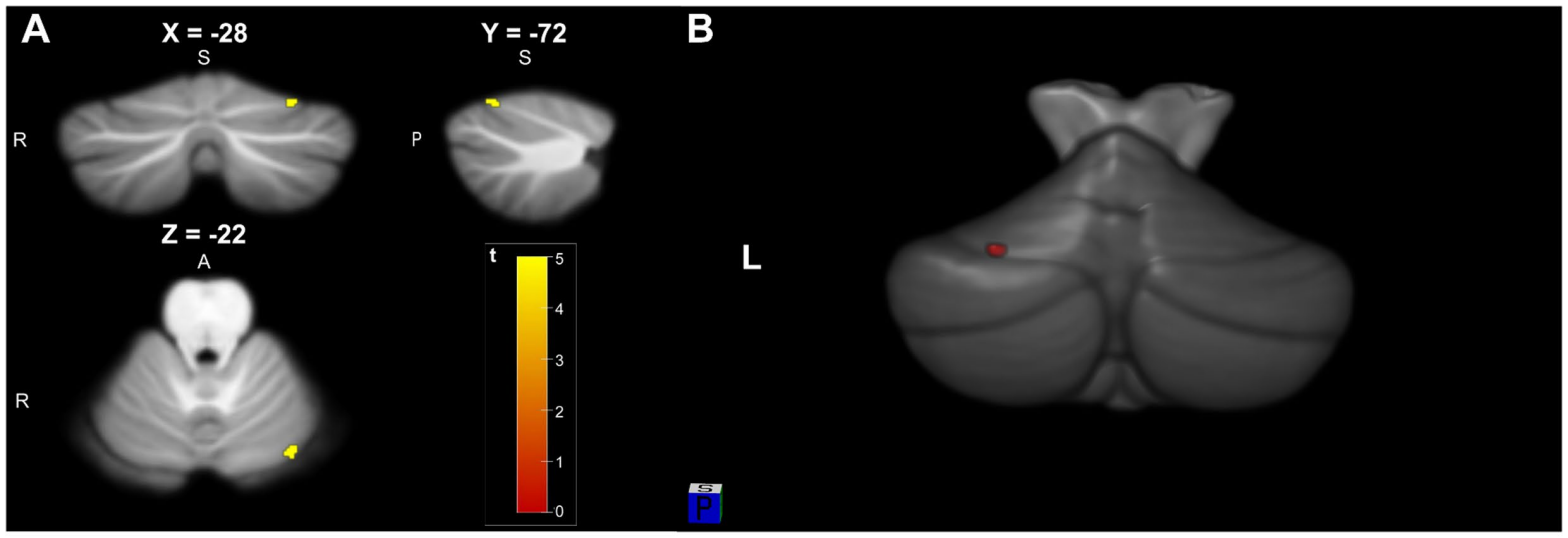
(A) Significant cluster of cerebellar gray matter volume reduction in Left lobule VI (−28, −72, −22) in TLE patients compared to HC (pFDR <0.05 and KE = 10). (B) 3D visualization model of cerebellar gray matter differential brain area superimposed on SUIT T1-weighted cerebellar template.
